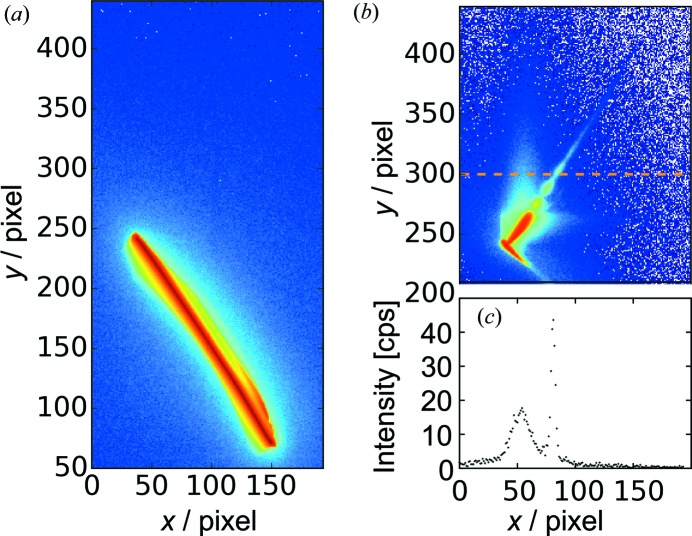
Detector images of (*a*) the direct X-ray beam (exposure time 20 s) and (*b*) the X-rays reflected from the sample (exposure time 10 s). (*c*) Intensity plot along the line *y* = 300 in (*b*).
